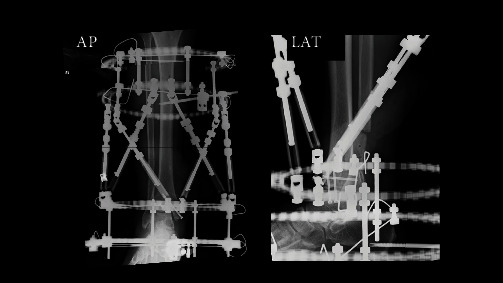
Post-surgery X-ray and circular external fixators. Rings were placed on the tibia at three locations, and a foot ring was placed on the foot at one location.
